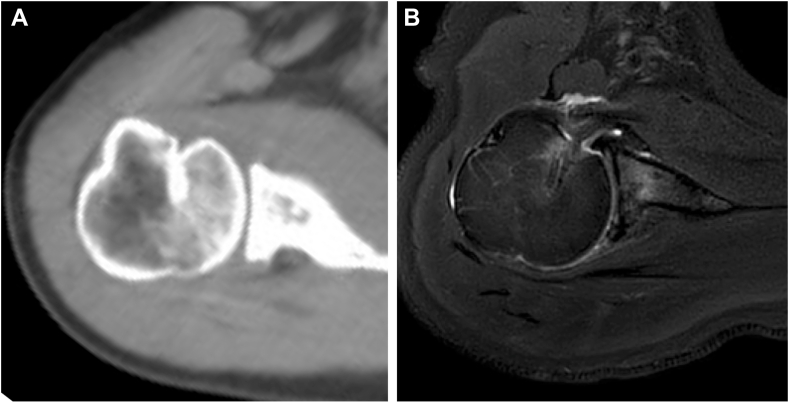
(A) Postoperative CT and (B) T2-weighted fat-suppressed MRI images of Case 2. CT scans were obtained 3 months after surgery, and MRI scans were acquired 6 months after surgery, confirming maintained reduction and successful attachment of the subscapularis to the reverse Hill-Sachs lesion. *CT*, computed tomography; *MRI*, magnetic resonance imaging.
